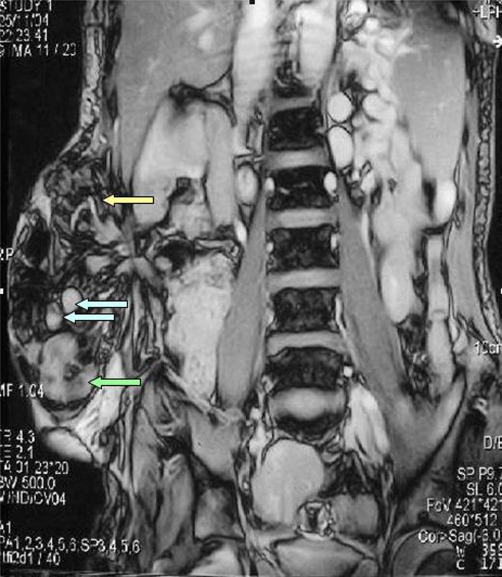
Magnetic resonance image of the abdomen showing a hernial sac containing gastric antrum (green arrow), segments of small intestine (blue arrow) and mesenteric fatty tissue (yellow arrow).
